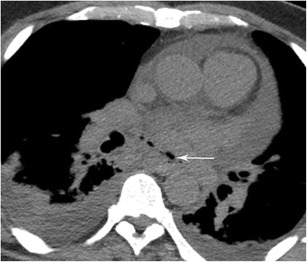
Atrioesophageal Fistula. CT of the chest in soft tissue window demonstrates thickening of the oesophageal wall with pockets of gas (arrow) within the left atrium. Additionally, there are bilateral pleural fluid collections and a pericardial effusion
